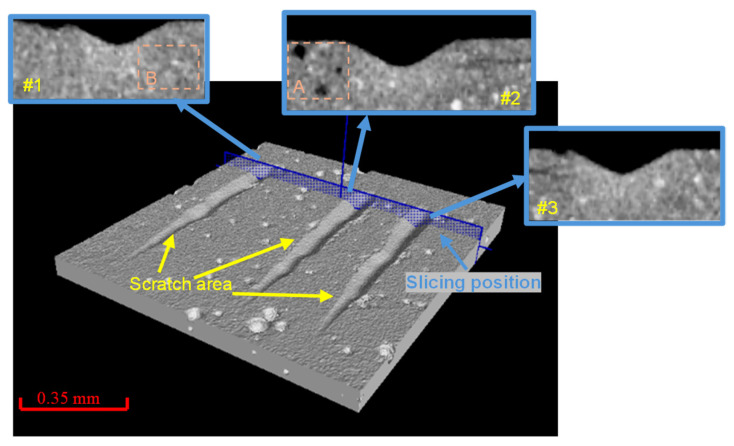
Reconstructed X-CT 3D image and 2D slice image along the scratch direction for the WCP scratch area.
